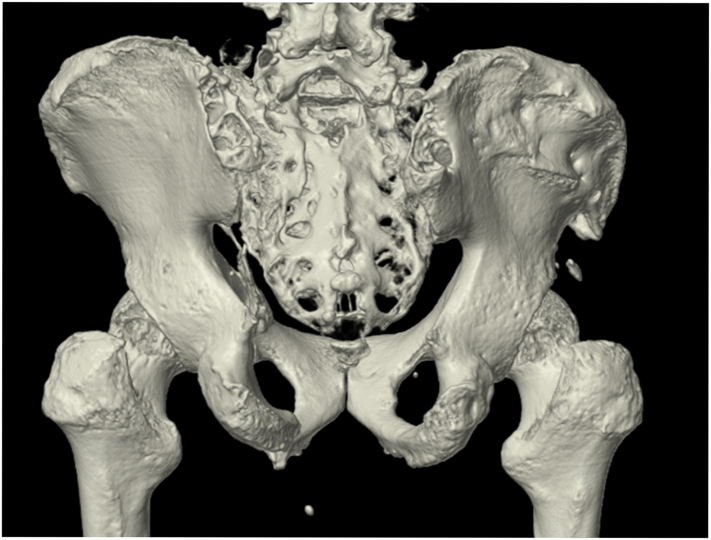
Computed tomography results at 2 years postoperatively showing appreciable bone fusion.
